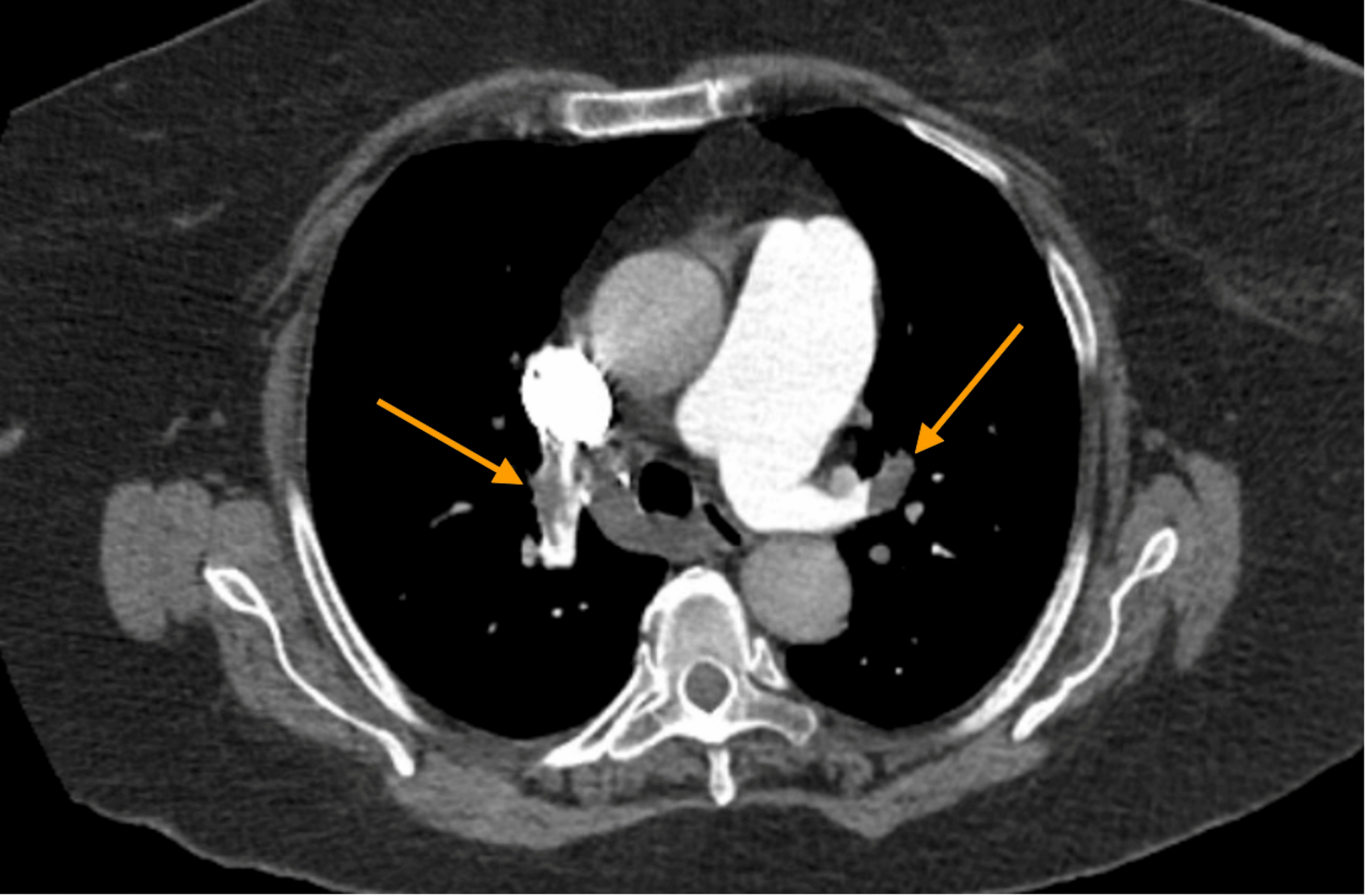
CT scan obtained at presentation demonstrating large proximal bilateral PE’s involving the lobar and segmental vessels. PE: pulmonary embolism.
